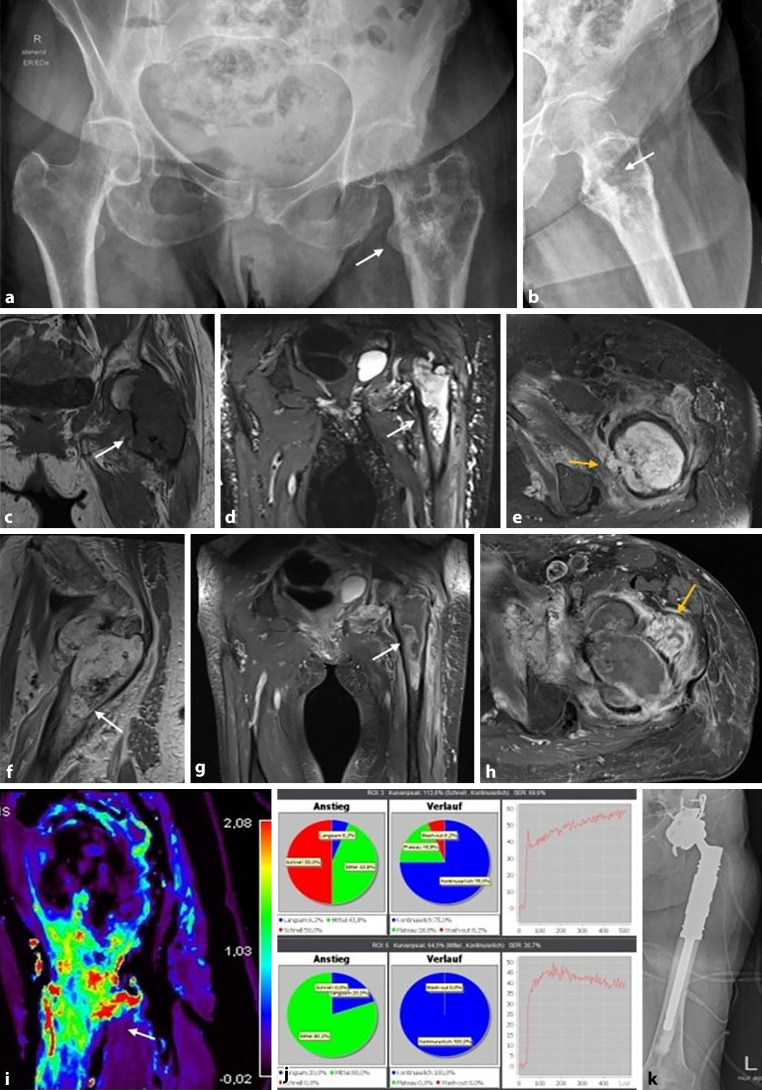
Case 3. AP radiography of the pelvis (a) and oblique projection of the left hip joint (b) show an osteolytic lesion in the trochanteric area with a cartilaginous matrix. On precontrast MRI, hypointense signal of the lesion (c) with cervical and diaphyseal extension of the tumor in the coronal T2-weighted image with a significant mucoid component. Axial T2-weighted image (e) with fat saturation shows the intraarticular extraosseous component of the tumor with surrounding bone marrow oedema on sagittal T2-weighted image (f). After contrast administration on coronal and axial T1-weighted images, nodular enhancement and typical lobular appearance of the tumor are shown (g, h). On dynamic contrast-enhanced MRI, diffuse patchy areas with high proliferation rate and time intensity curve Type 4 and Type 5 (i, j). Postoperative radiography of the lower extremity with tumor hip endoprosthesis (k)
